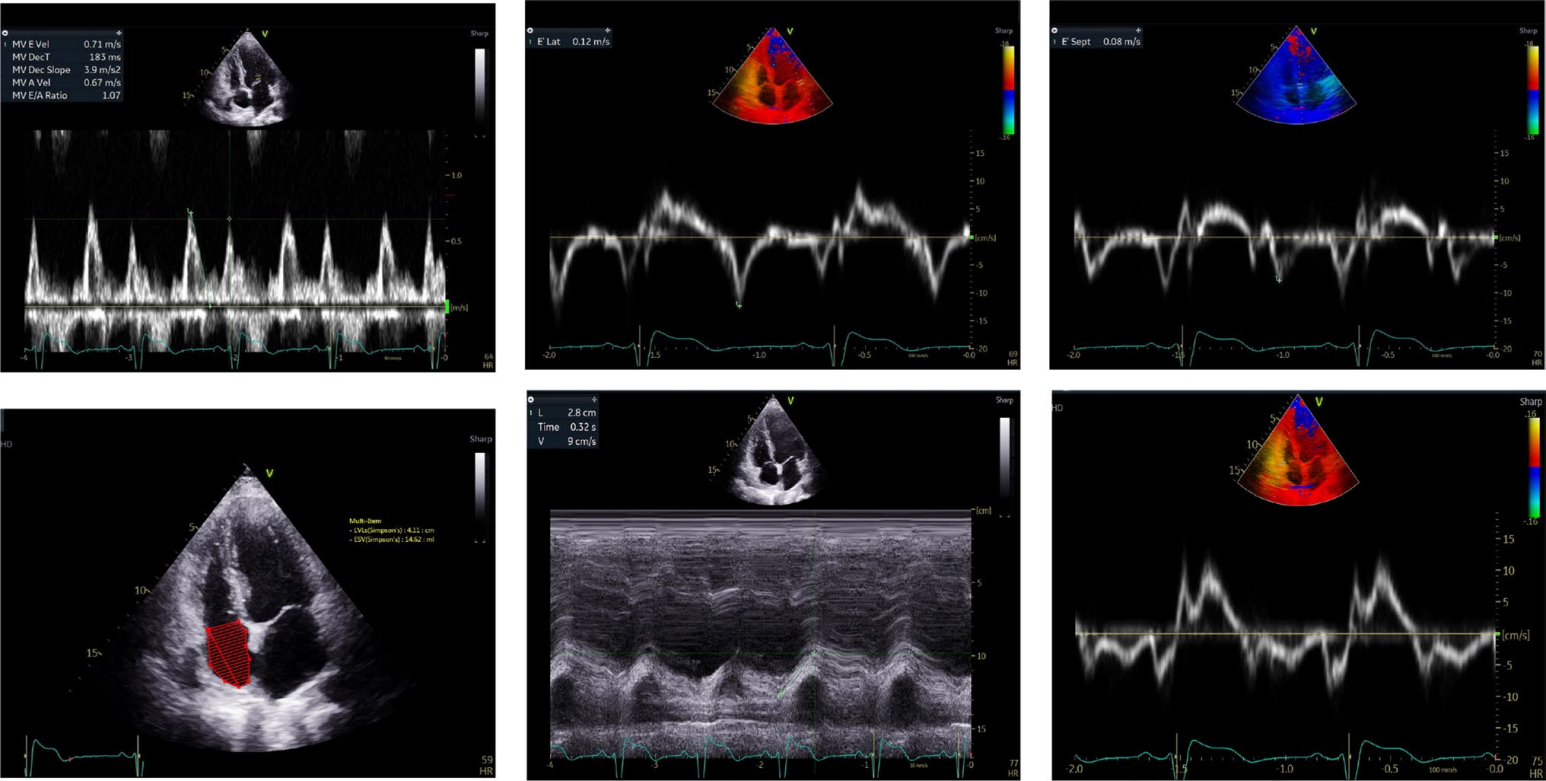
Echocardiographic Assessment of studied patients with CAT score <10
